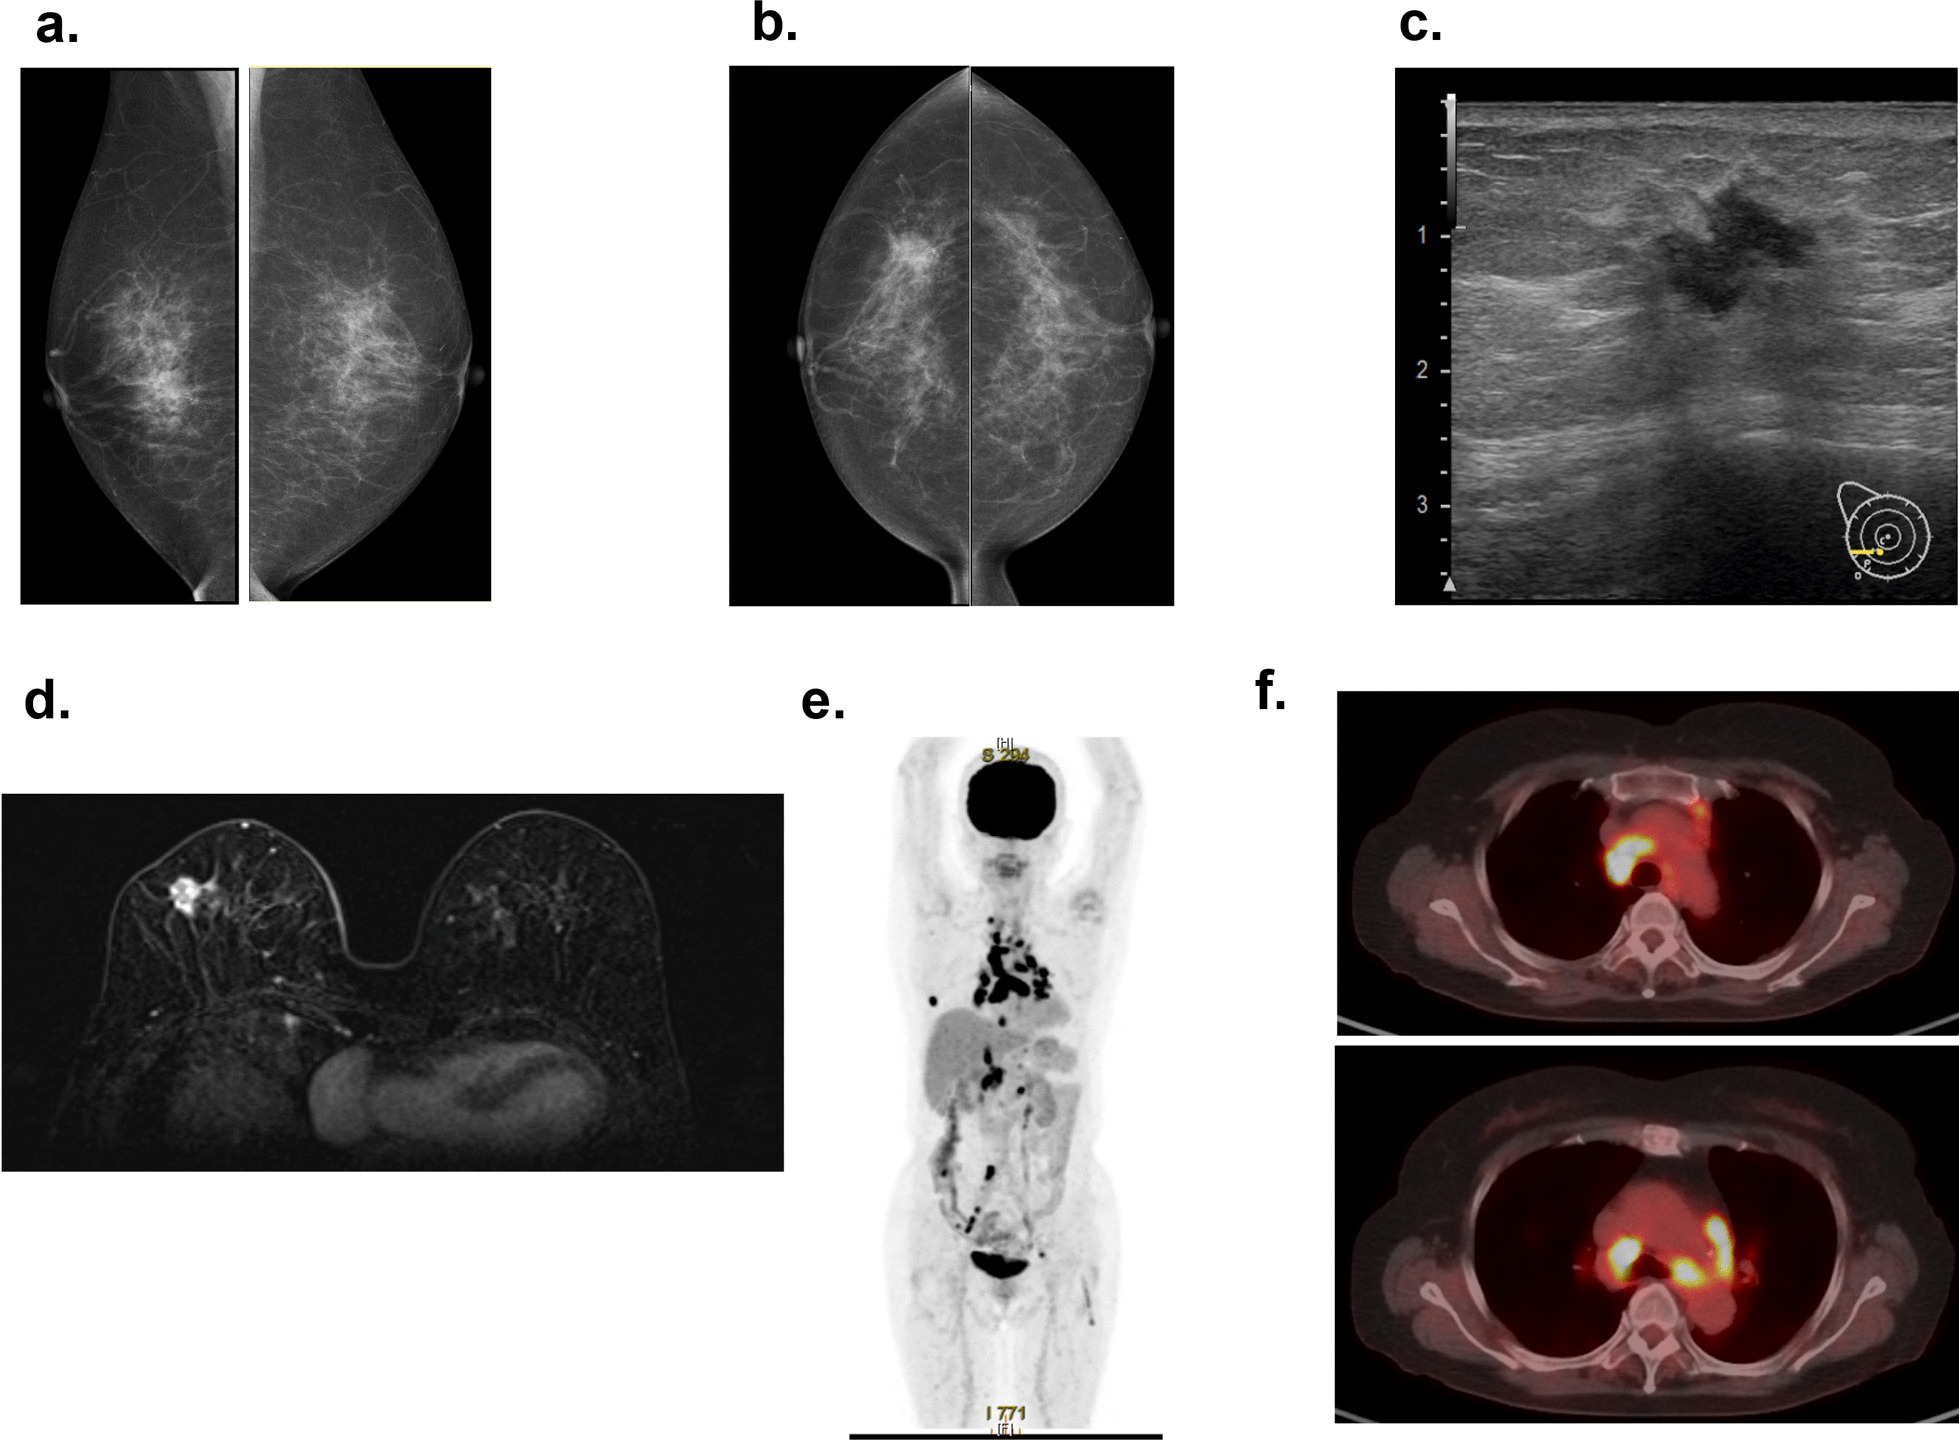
Preoperative imaging findings. a Mediolateral oblique-view mammogram. b Craniocaudal-view mammogram. A spiculated mass (A) with segmental and linear calcification is recognized in the right breast. c Ultrasonogram. A rough-bordered mass with internal hypoechogenicity is apparent in the inferolateral quadrant of the right breast. d MRI with early gadolinium enhancement. A smooth, round mass with rim enhancement is recognized in the inferolateral quadrant of the right breast. e Positron emission tomography. Accumulation of FDG is evident in the primary mass in the right breast, a right axillary lymph node, and multiple lymph nodes in the right supraclavicular fossa, mediastinum, bilateral hilar, dorsal pancreatic head, abdominal aorta, right common iliac region, and bilateral external iliac regions. f Positron emission tomography/computed tomography. Intense FDG uptake is shown in enlarged paratracheal lymph nodes
